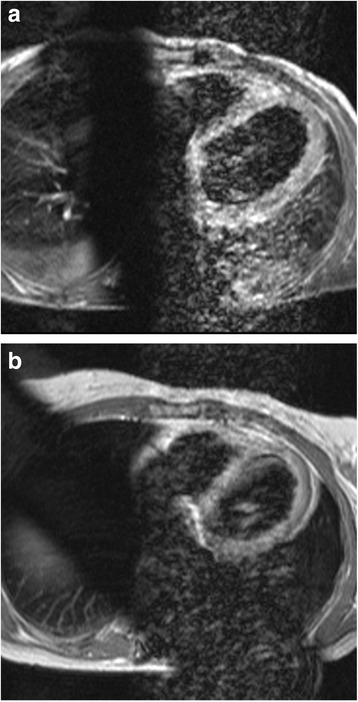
Time course of cardiac magnetic resonance imaging. a 23 December 2014: cardiac magnetic resonance four-chamber view showing relative enhancement of the left ventricle post-contrast media administration with an elevated T1-ratio of 9.05 (normal range <4) indicating hyperemia and capillary leakage. b 3 June 2015: cardiac magnetic resonance four-chamber view showing a normal T1-ratio of 2.25 (normal range <4)
